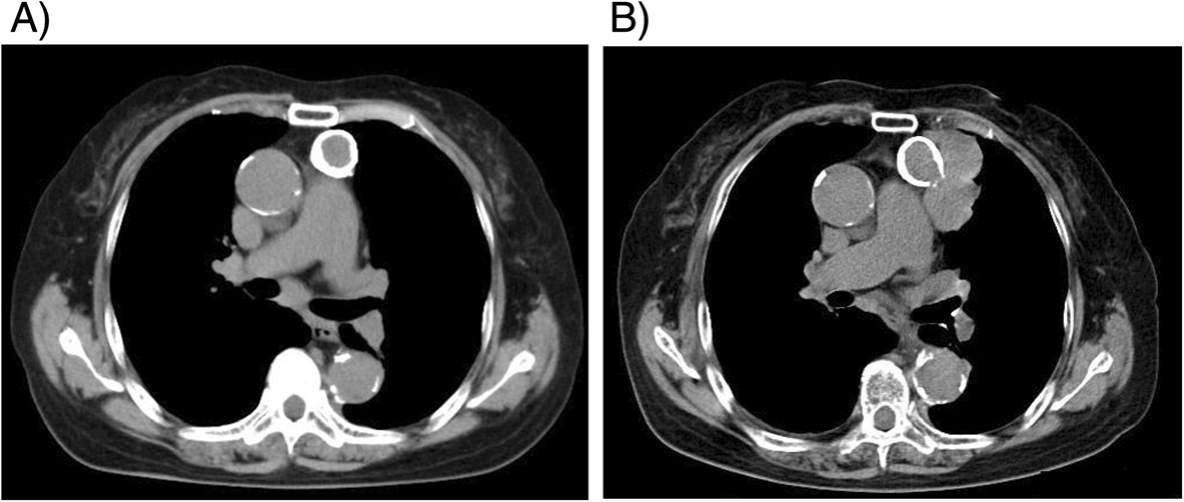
Chest computed tomography of the anterior mediastinal tumor a was taken when the tumor was detected for the first time. b Tumor growth after 4 years. The tumor grew outside the calcification and adjacent to the left lung
